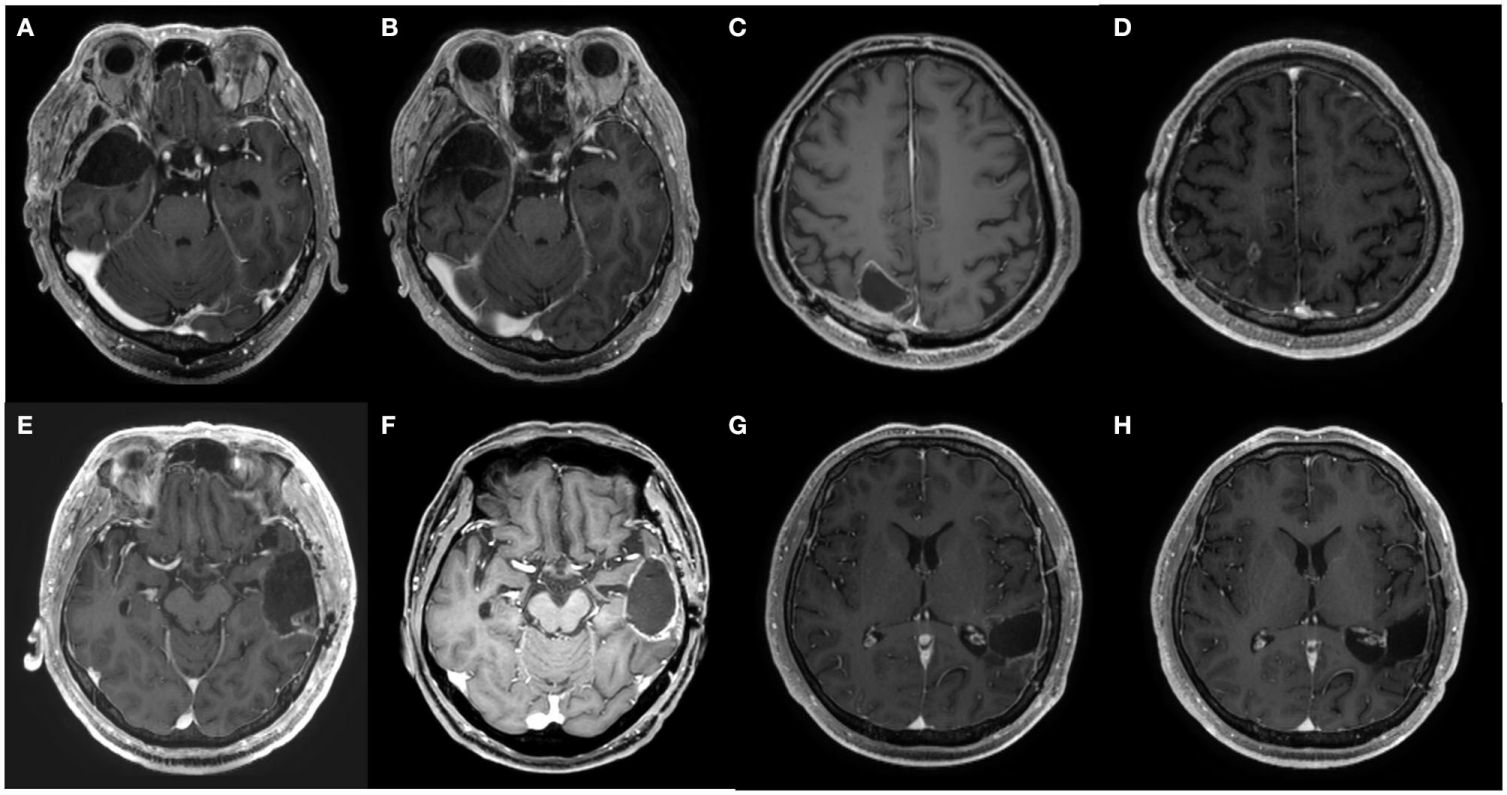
Axial MRI images at pretreatment and at 1 year after the chemotherapy of patients 2, 3, 4, and 5. (A,B) Pre- and post-treatment MRI of patient 2, respectively. (C,D) Pre- and post-treatment MRI of patient 3, respectively. (E,F) Pre- and post-treatment MRI of patient 4, respectively. (G,H) Pre- and post-treatment MRI of patient 5, respectively.
